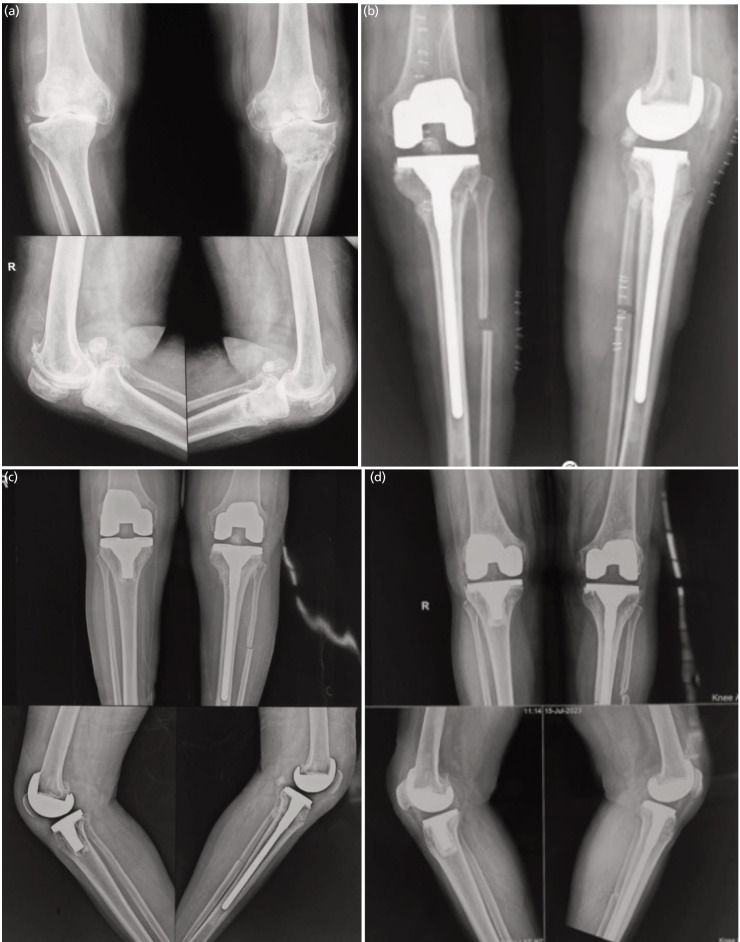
(a) Radiographs showing severe osteoarthritis of both knees with chronic stress fracture on left side with non-union. (b) Post TKA radiograph showing long tibial stem implant and Fibulectomy. (c) One-year follow-up Radiographs. (d) Two years follow-up radiographs showing complete union of tibia.
